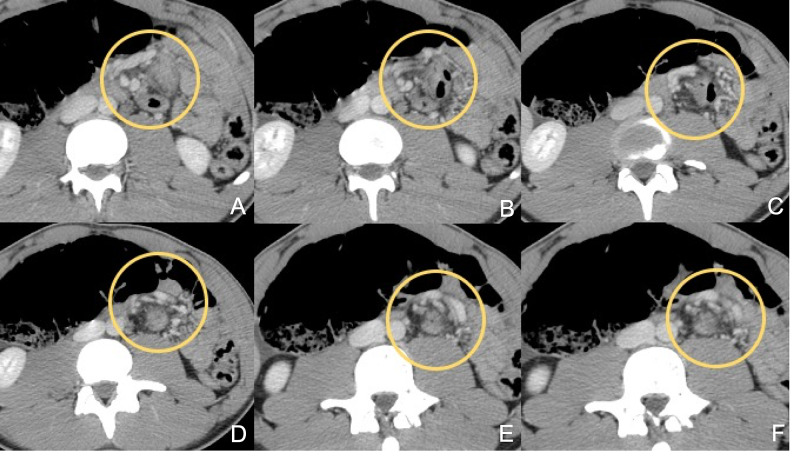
CECT view 1 Progressive axial slices showing the ‘whirlpool’ sign (yellow circle) CECT: contrast-enhanced computed tomography
